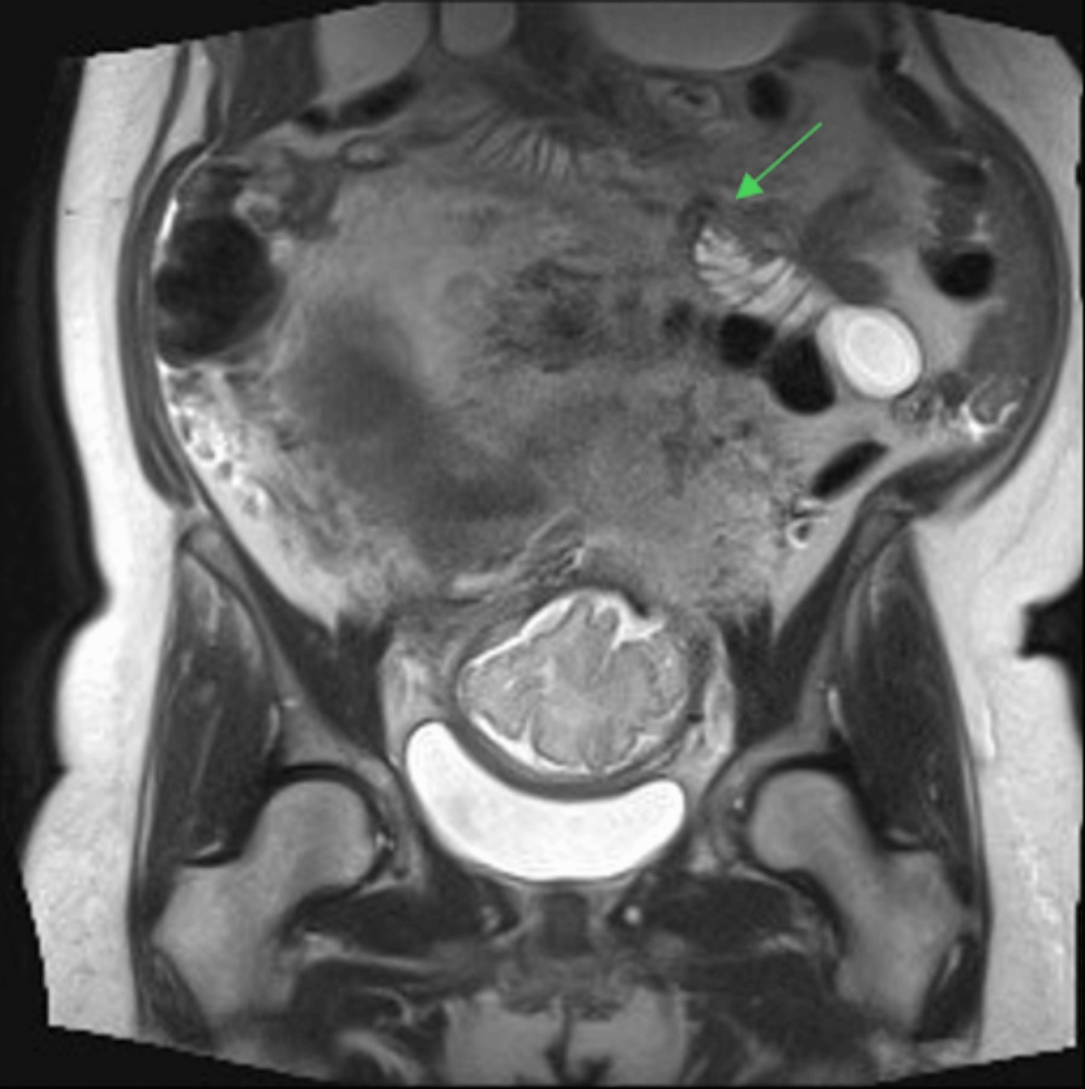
MRI T2 coronal plane demonstrating transition point from dilated to non-dilated proximal bowel loops approximately 20 cm distal to the duodenojejunal flexure
